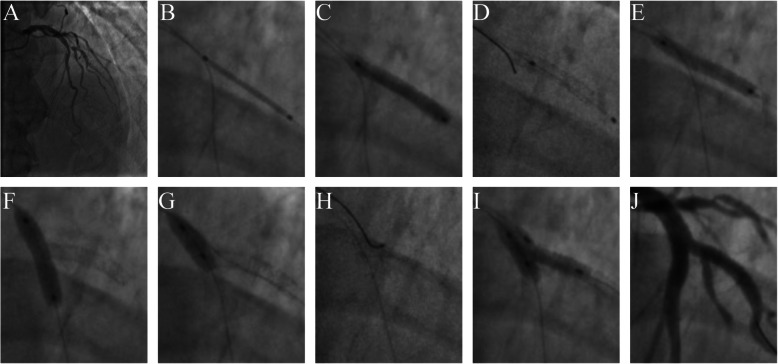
Step-by-step Szabo 2-stent technique application in a patient. Baseline coronary angiogram was presented in (a). Diagonal stent is precisely positioned (b) (see Online Video 6) and deployed (c). LAD rewiring free from strut (d) (see Online Video 7) and optimizing diagonal stent (e) results in one strut protrusion into LAD (see Online Videos 8 and 9). LAD stenting (f) and optimization (g) followed by diagonal branch rewiring (h) (see Online Video 10) and final kissing inflation (i) (see Online Video 11). Final result (j) is assessed by coronary angiography (j) and intravascular ultrasound (see Online Videos 12 and 13) to confirm the struts gap and overlap. LAD = left anterior descending artery
